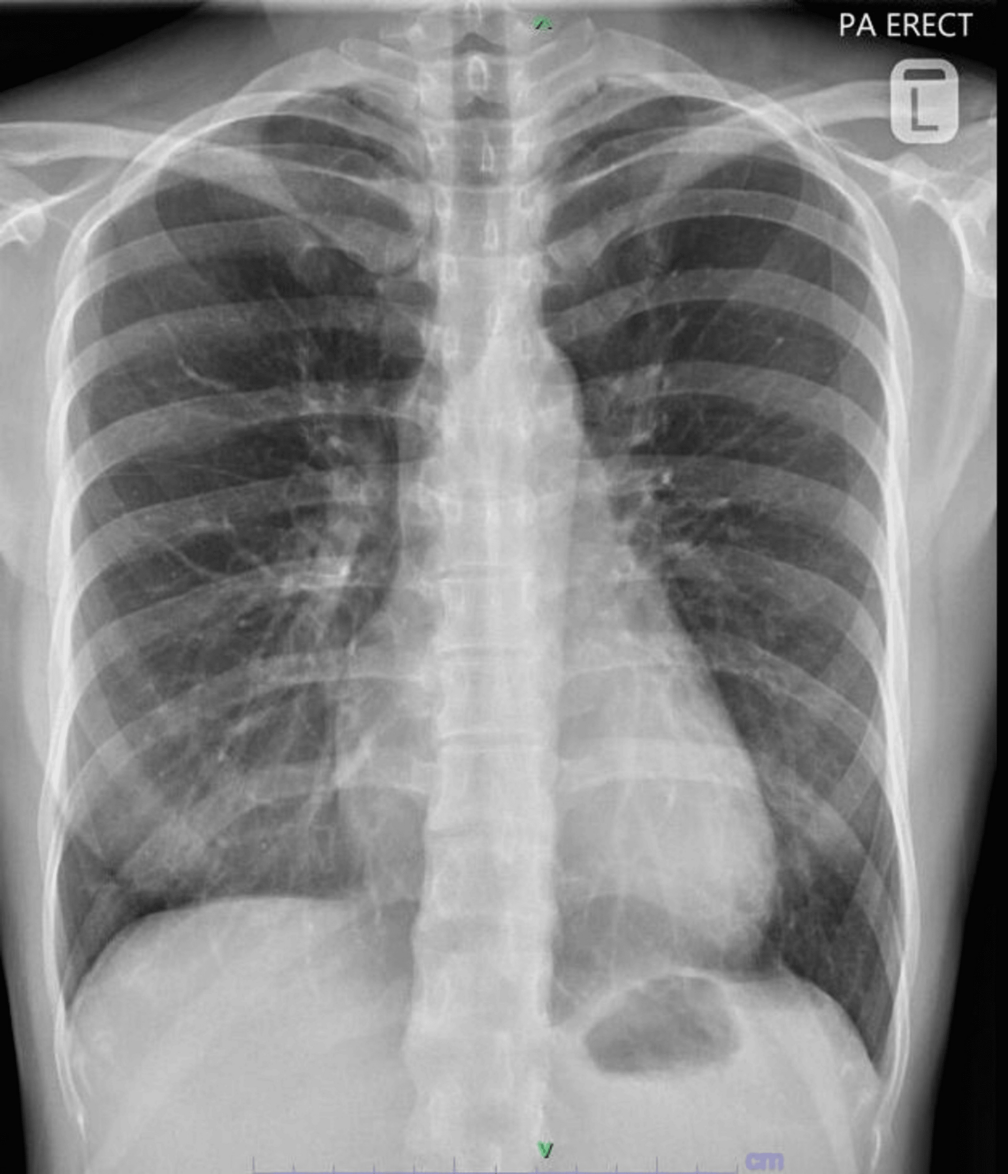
Chest radiograph (posteroanterior erect). The image obtained preoperatively shows the resolution of pneumothorax.
